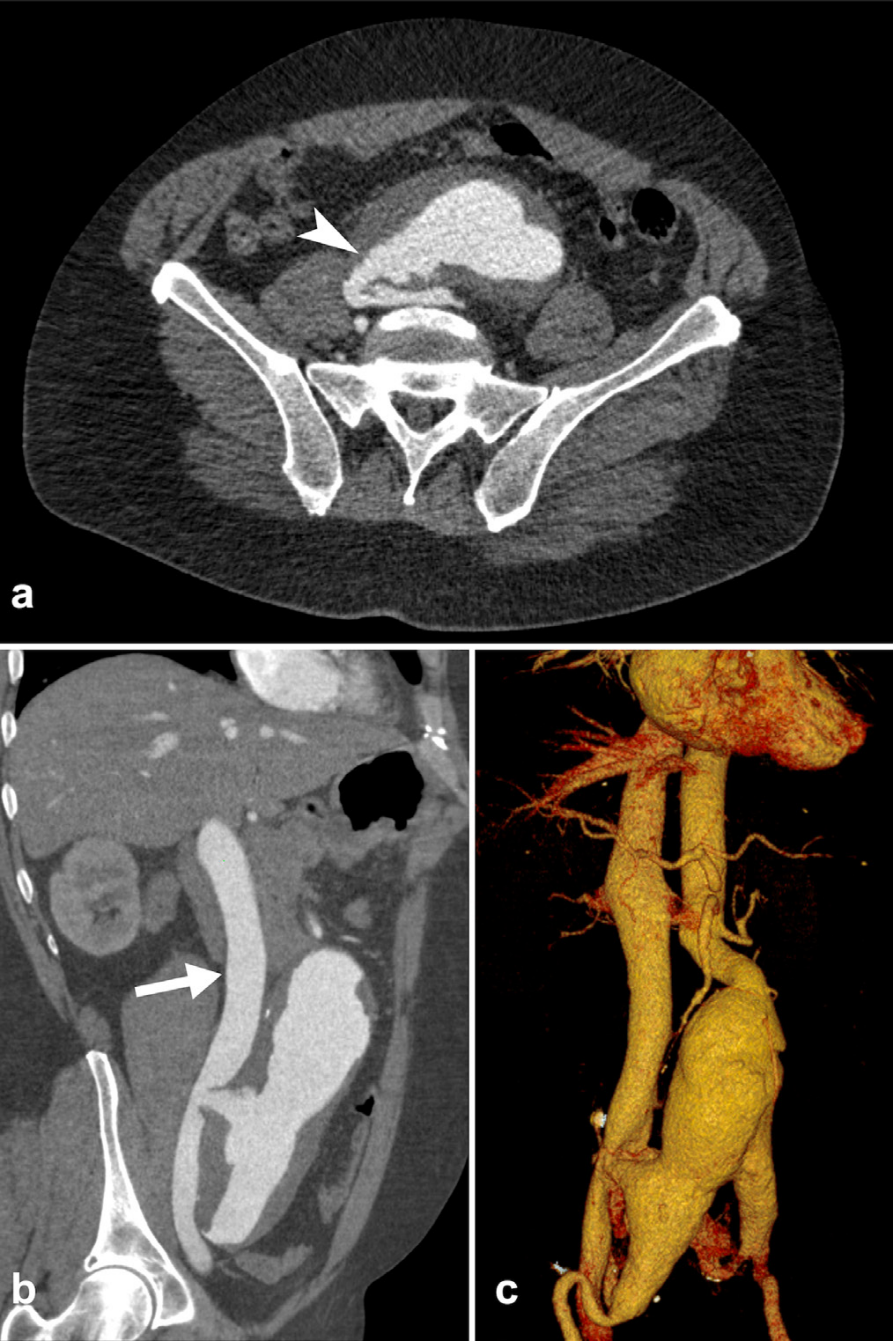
Contrast-enhanced CT scan during arterial-phase showing the presence of a fistula between the aortic aneurysm and the IVC. (a) Axial images well depict the communication between the two vessels (arrowhead). (b, c) MPR and VRT reformatted images show the early full enhancement of the dilated IVC (arrow) during the arterial phase
